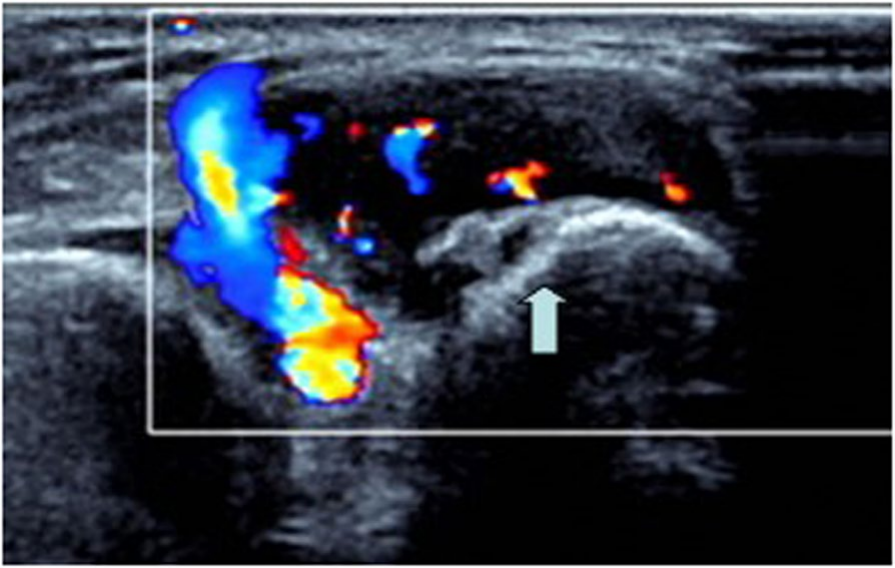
Axial sonogram of the index finger shows mild scalloping (arrow) of the flexor surface of the proximal phalanx
